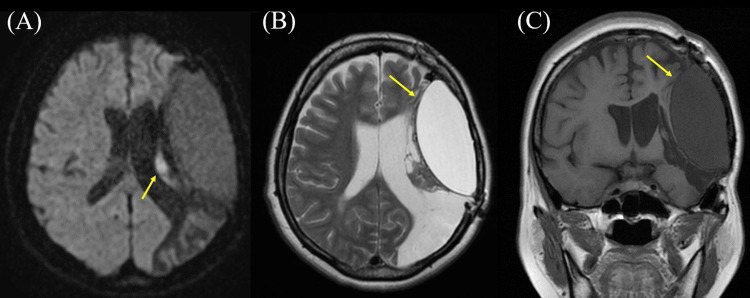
The result of brain magnetic resonance imaging (MRI). (A) Diffusion-weighted brain MRI on admission showing hyperintensity in the left corona radiata near the double skull sign (DSS) (arrowhead). (B) T2-weighted brain MRI (axial section) 2.5 months after admission showing hyperintensity inside DSS (arrowhead). (C) T1-weighted brain MRI (coronal section) 2.5 months after admission showing hypointensity inside DSS (arrowhead).
